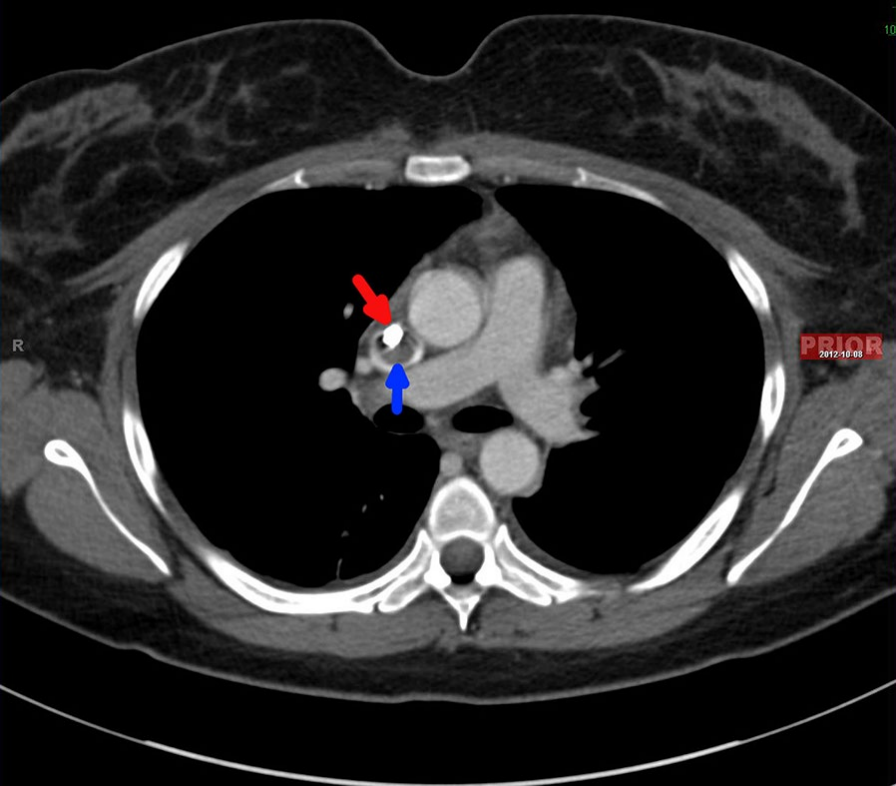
CT chest with contrast (*transverse view*) demonstrating an HD catheter (*bright white* shown by *red arrow*) with surrounding thrombus in the superior vena cava (*blue arrow*)
